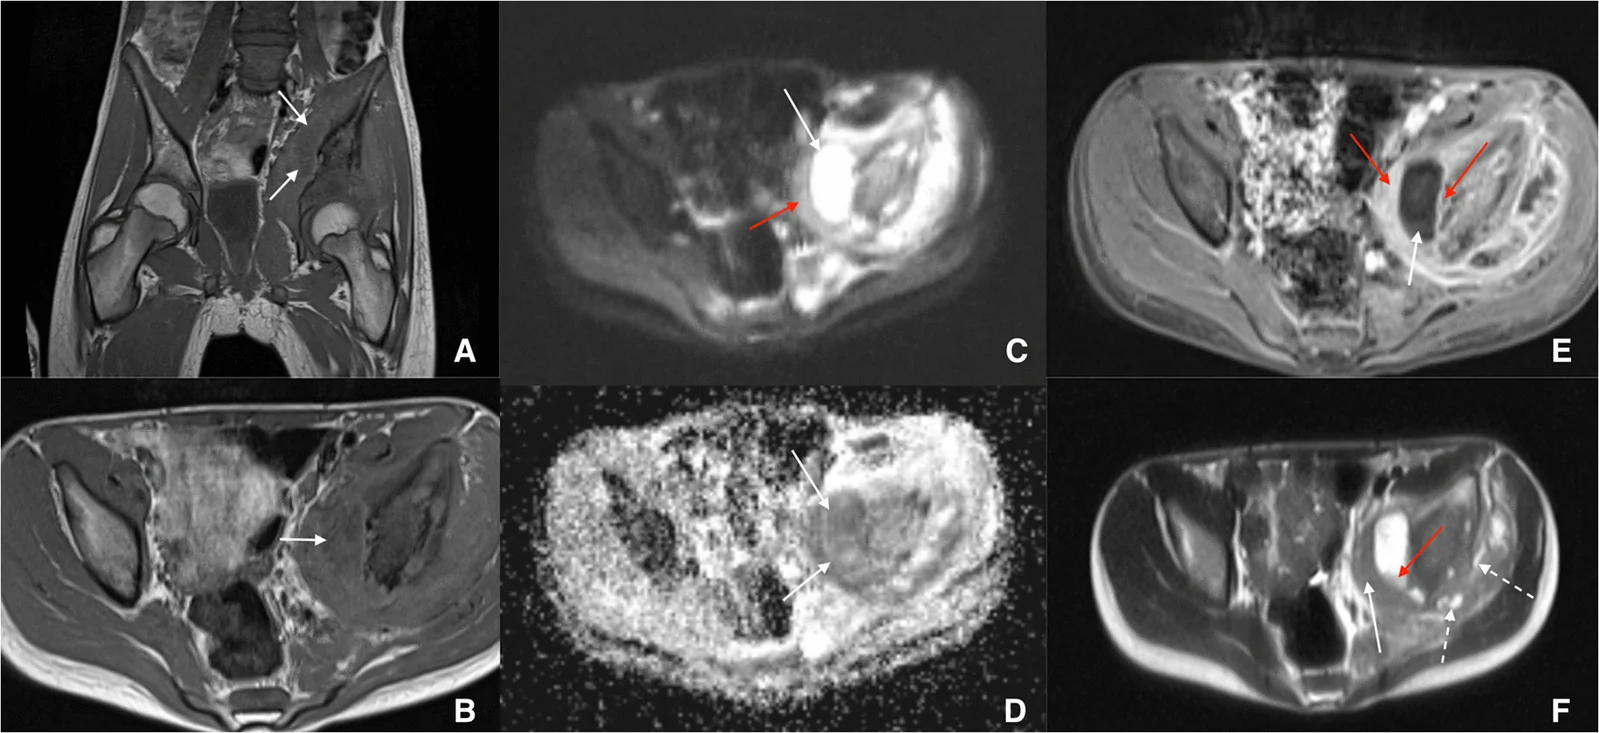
Coronal (A) and axial (B) T1-weighted MRI of the pelvis showed a hypointense soft-tissue and marrow-replacing lesion sparing the pelvic and triradiate cartilage (white arrows). Axial diffusion-weighted imaging suggested marked diffusion restriction (white arrow) within adjacent rim-enhancing soft-tissue collections (red arrow), consistent with abscess formation (C). ADC mapping confirms restricted diffusion with low-signal in the respective bone (white arrows) (D). Axial postcontrast T1 fat-suppressed images demonstrate medial and lateral rim-enhancing fluid collections (red arrows) adjacent to the lesion (white arrow), compatible with abscesses, with surrounding inflammatory enhancement (E). Axial T2 (F) imaging characterized extensive marrow, soft-tissue, and fascial edema surrounding the lesion (white arrow) with a focal fluid collection (red arrow) and smaller lateral abscesses (dashed arrows)
